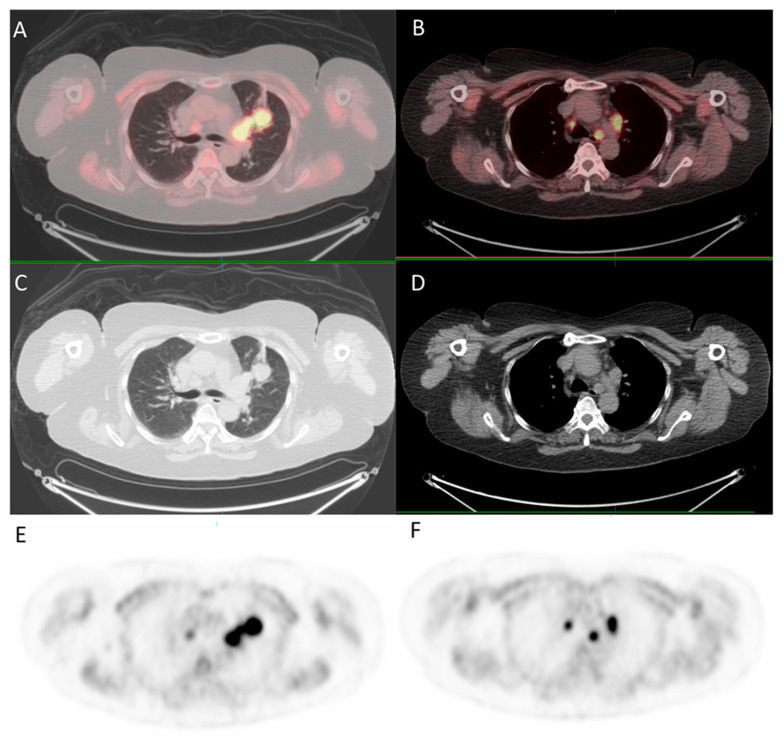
^18^F-fludeoxyglucose positron emission tomography/computed tomography (^18^F-FDG PET/CT) for whole-body evaluation. (A,B) axial fused PET/CT showing focal uptake of the lung and mediastinum, respectively; (C,D) axial CT; (E,F) PET.
